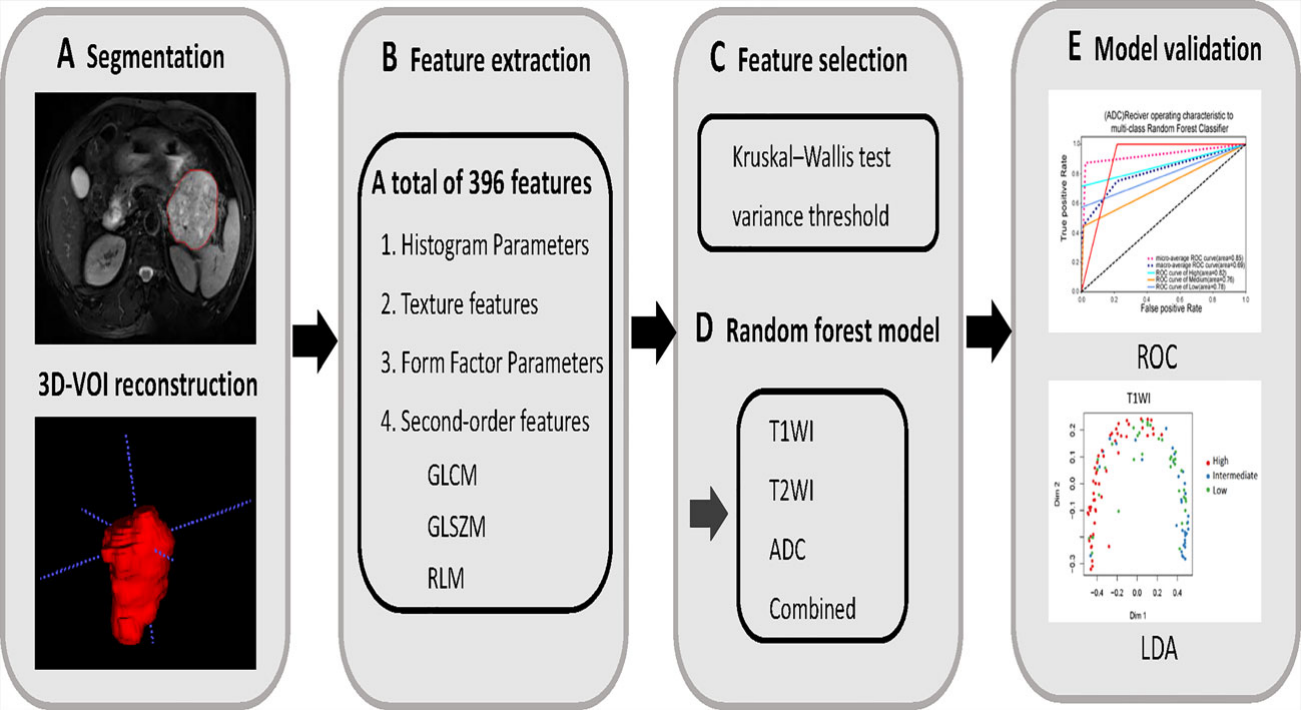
(A) MR images segmentation. The ITK-SNAP software was used to manually outline and segment the tumor region by slices, and then 3D-VOI reconstruction was performed to extract the texture parameters. (B) Texture features extraction. According to the segmentation image, a total of 396 texture parameters of different risk of GISTs were extracted from each set of images. (C) Texture features selection. After the parameters were normalized and dimensionality reduced, the characteristic parameters were selected by Kruskal–Wallis test and variance threshold. (D, E) Model establishment. Using random forest to build multi-sequence MRI model and its diagnostic efficacy was evaluated by ROC and LDA analysis.
